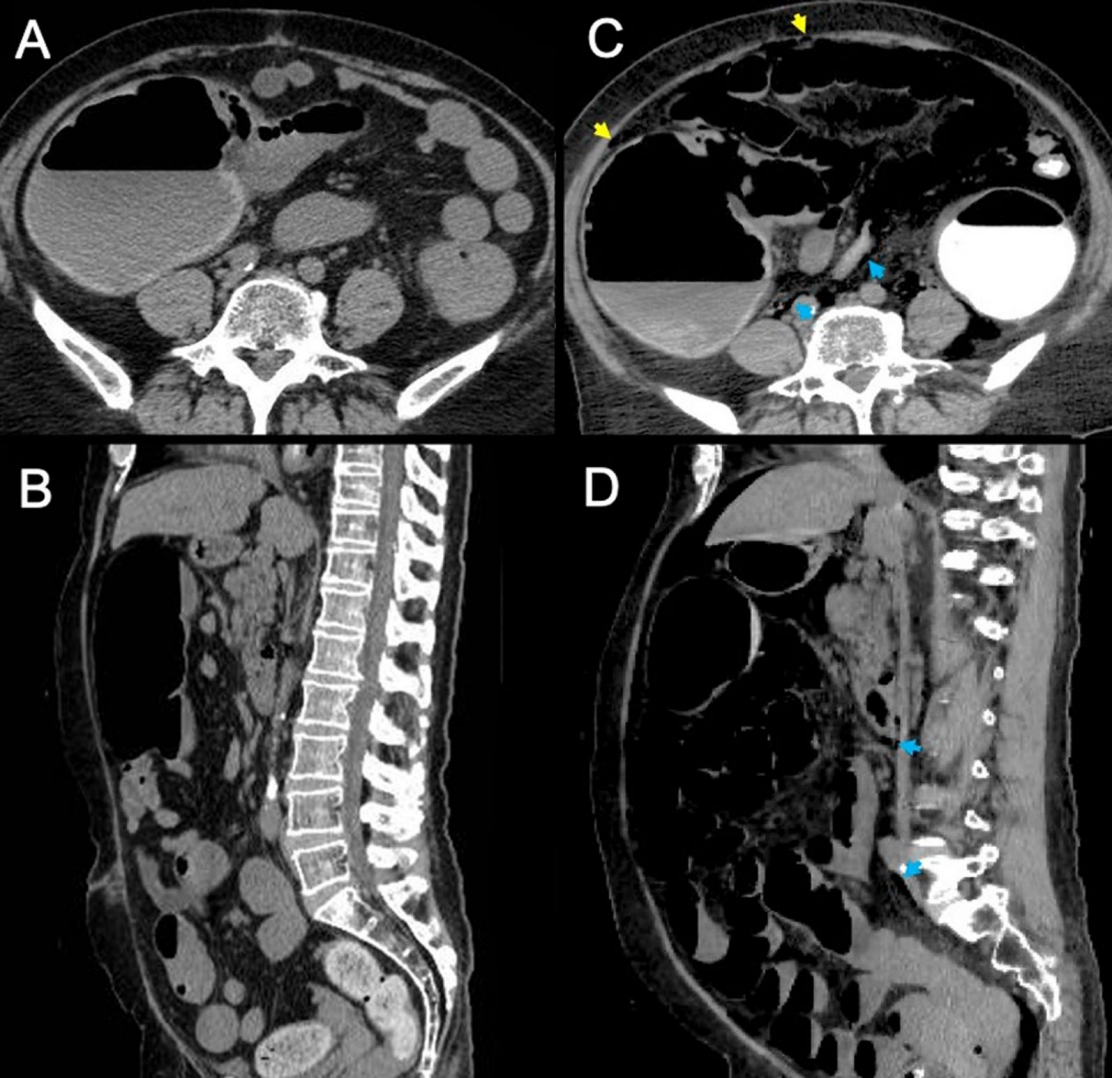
Computed tomography scans obtained after and before colonic stenting. a An axial view before colonic stenting. b A sagittal view before colonic stenting. c An axial view after colonic stenting shows the presence of air in the abdominal cavity (yellow arrows) and retroperitoneal cavity (blue arrows), mainly in the mesentery of the sigmoid colon, and dilatation of the colon without spillage of contrast. d A sagittal view shows marked distention of the abdomen caused by accumulation of gas in the small intestine and colon
